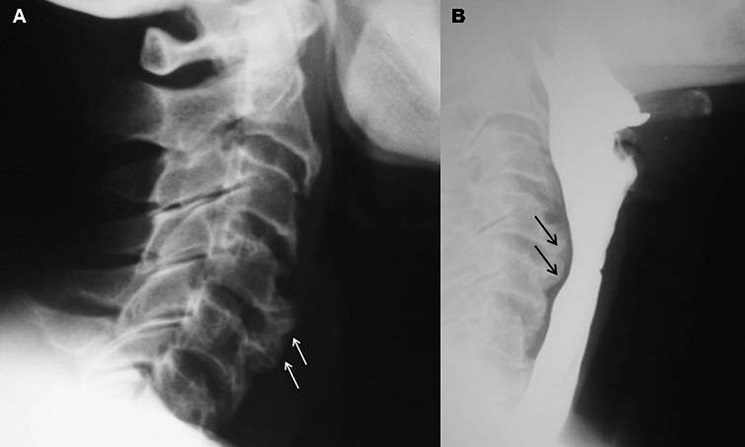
A) Radiographie du rachis cervical de profil: ostéophytes cervicaux antérieurs C4-C5 et C5-C6 (flèches blanches); B) Transit oesogastroduodénal: compression de la paroi postérieure de l'œsophage en regard des ostéophytes (flèches noires).
